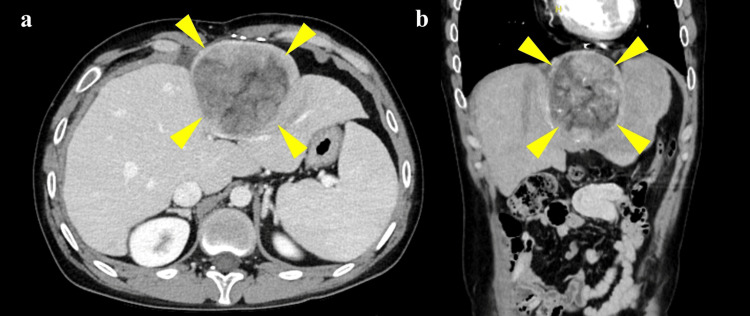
Contrast-enhanced abdominal computed tomography (CT) revealing a massive liver tumor (a) Axial and (b) coronal contrast-enhanced CT images showing a massive liver tumor (approximately 8.3 cm in diameter; yellow arrowheads) occupying the left lobe of the liver.
